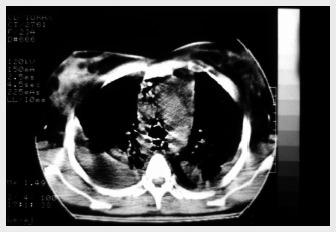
Chest computed tomography scan showing widening of mediastinal region and pleural effusion.
